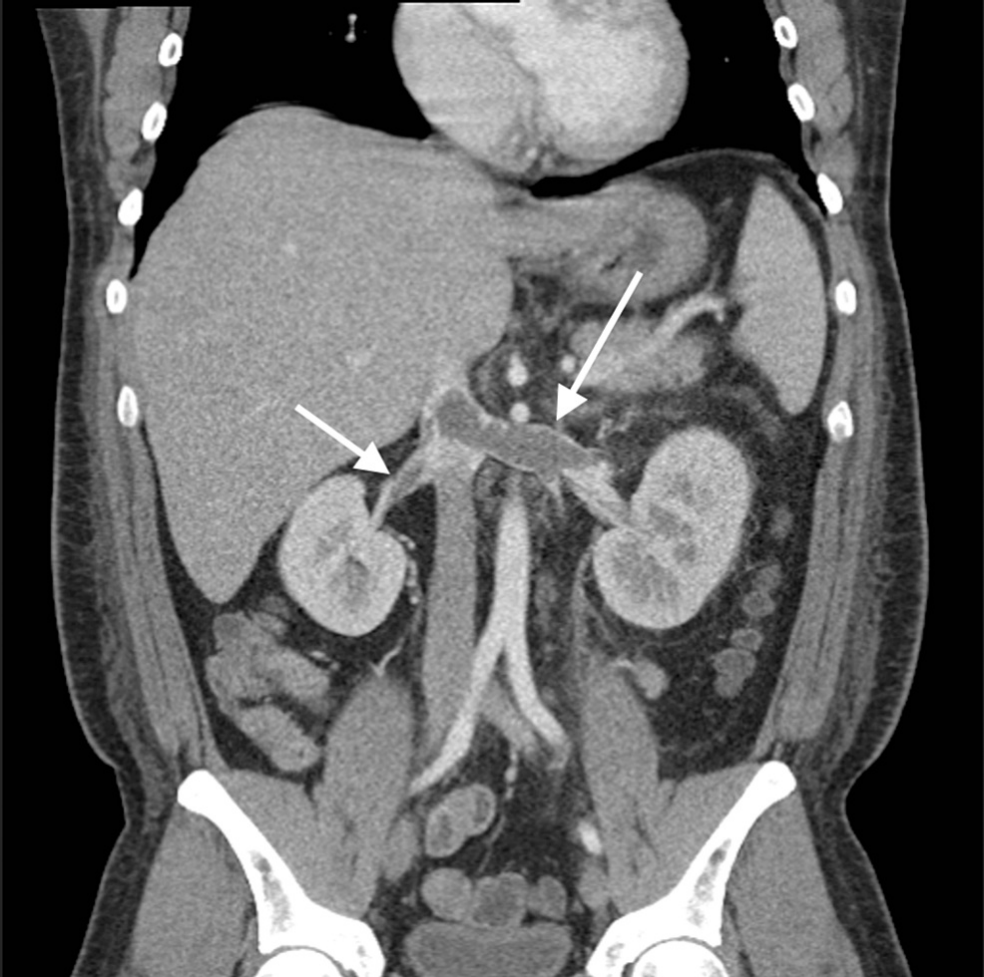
CTPA Demonstrating Bilateral Non-Occlusive Renal Vein Thromboses CTPA: computed tomography pulmonary artery
